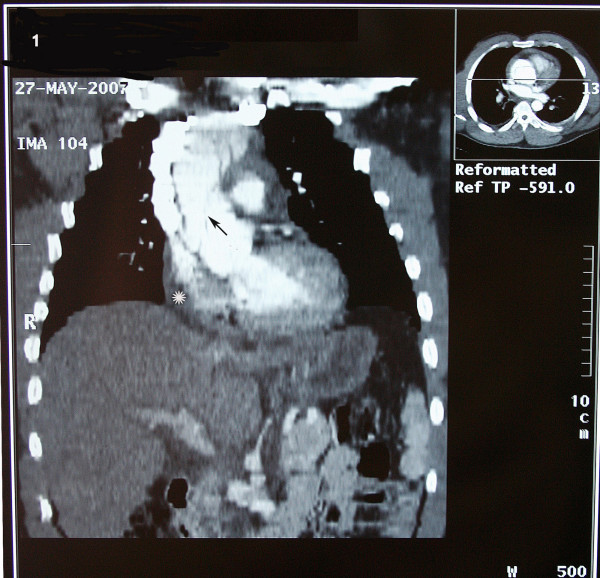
Semicoronal reconstructed CT angiography reveals flap in the ascending aorta and arch (arrow). The false and true lumens are patent. Hemopericardium is also seen (astrix).
